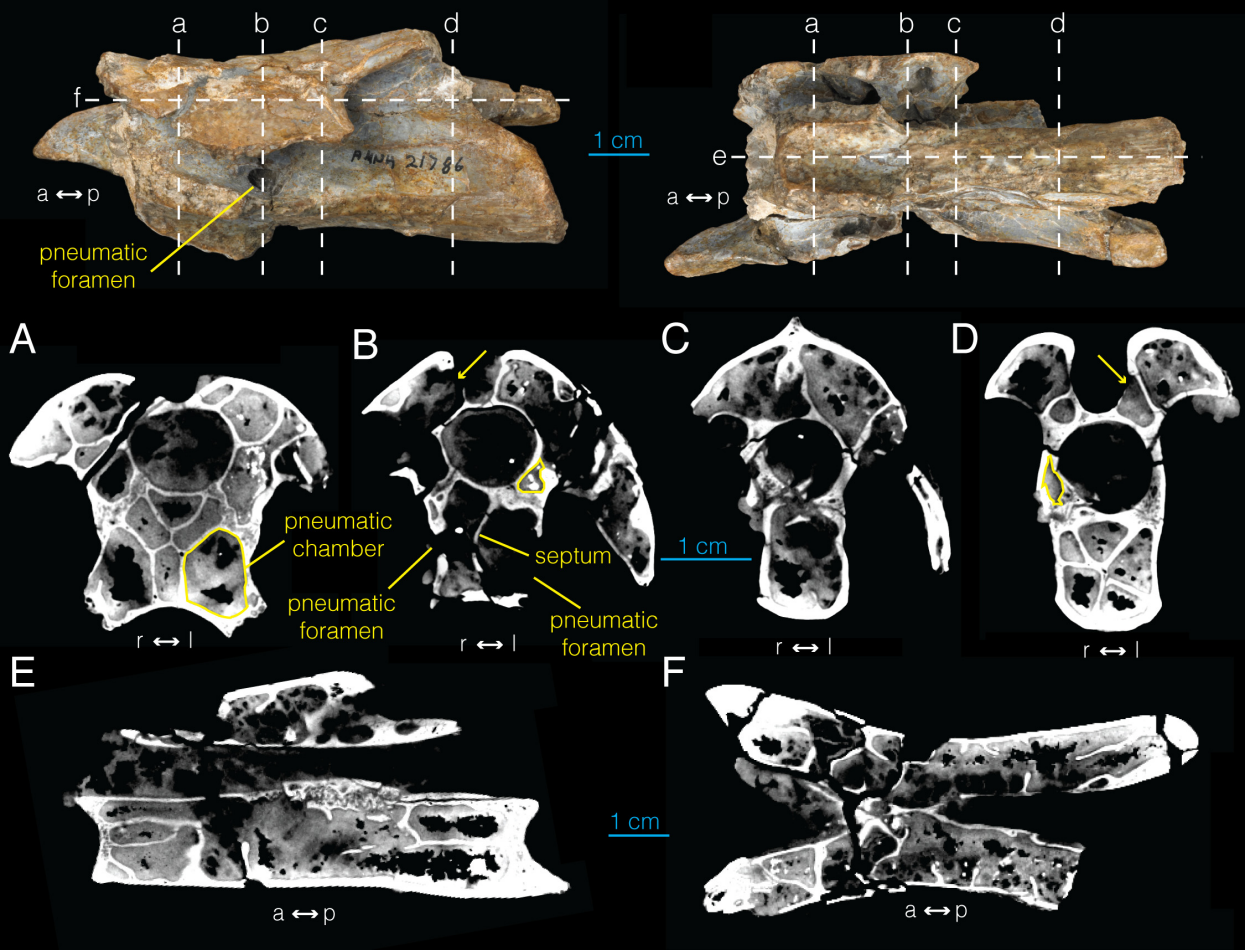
CT images of postaxial cervical vertebra of *Archaeornithomimus* (AMNH FARB 21786). A–D, select transverse sections; E, midsagittal section; F, frontal section. Dashed lines and associated letters indicate location and letter designation of CT image slices.
